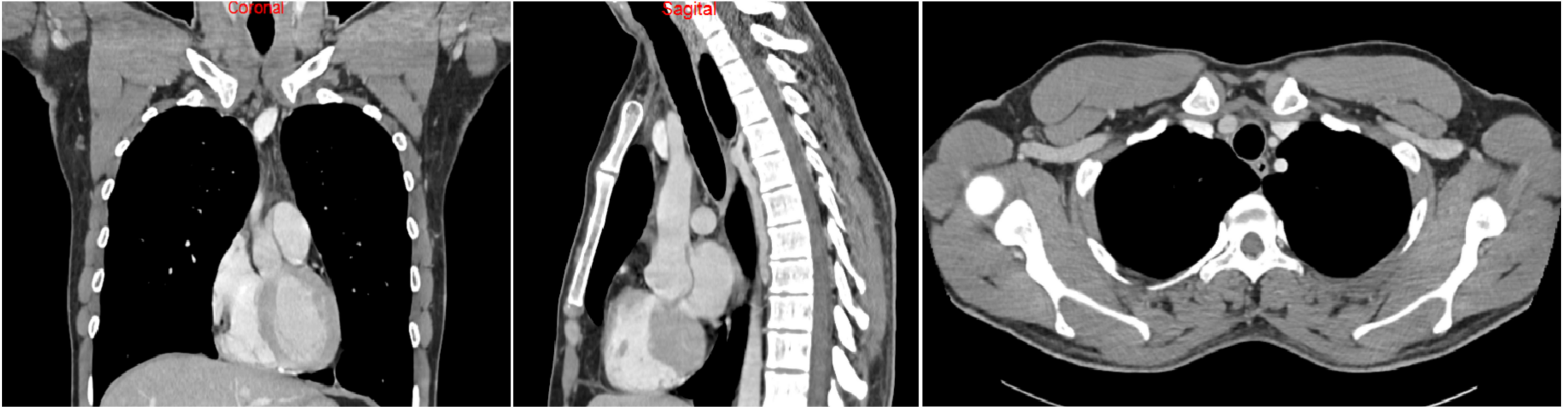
CT scan demonstrates complete response under of local and lymph node recurrence treatment with lorlatinib.
